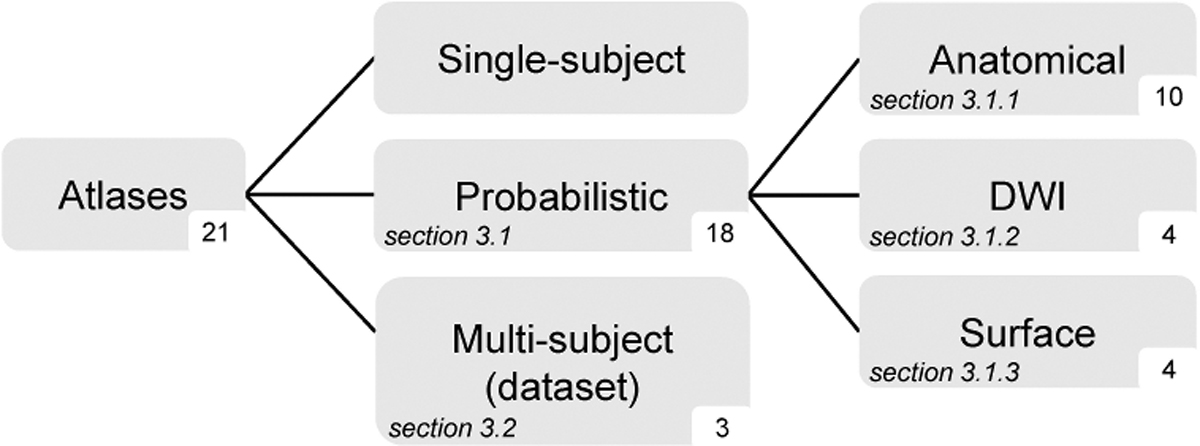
Categorization of atlases. The number of atlases per category is reported at the bottom right of each box. No single-subject atlas has been identified. Probabilistic atlases are categorized on the basis of the reference image used to characterize the brain anatomy (i.e., anatomical, diffusion, and surface images). DWI: diffusion weighted imaging.
